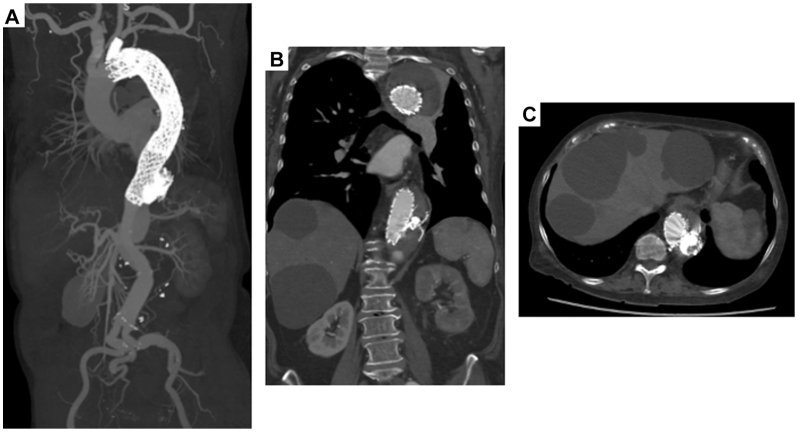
Contrast-enhanced computed tomography (CT) obtained at 3 weeks shows no migration or displacement of the candy-plug (A) and complete thrombosis of the thoracic false lumen (FL) (B and C).
